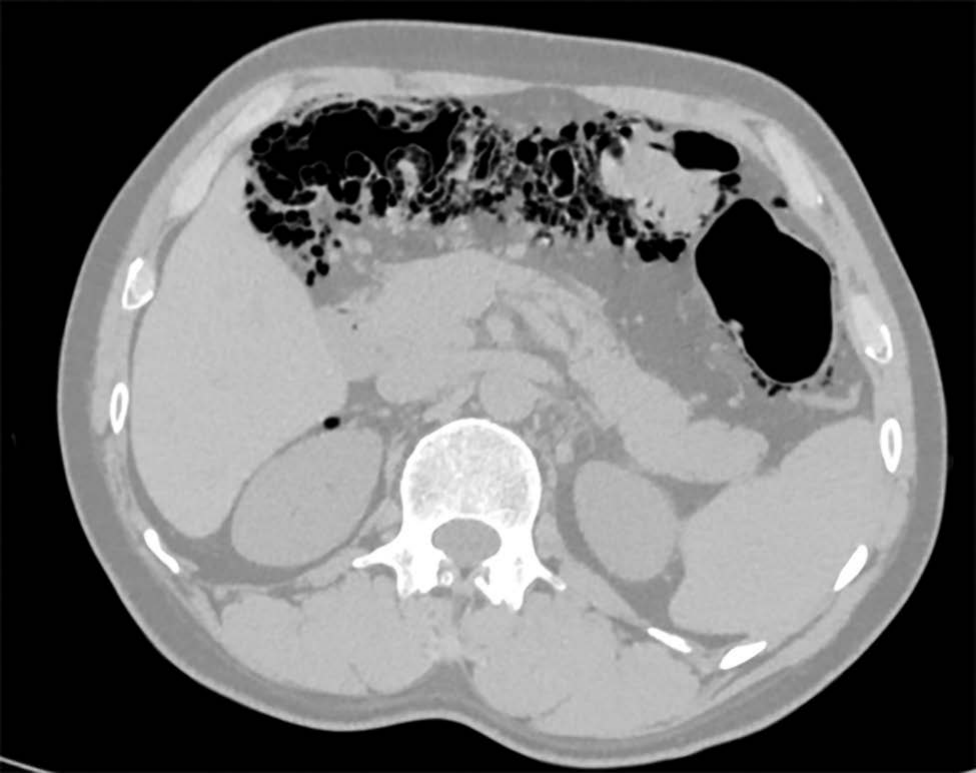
June 2, 2025, CT revealed PCI with pneumoperitoneum. CT = computed tomograph, PCI = pneumatosis cystoides intestinalis.
